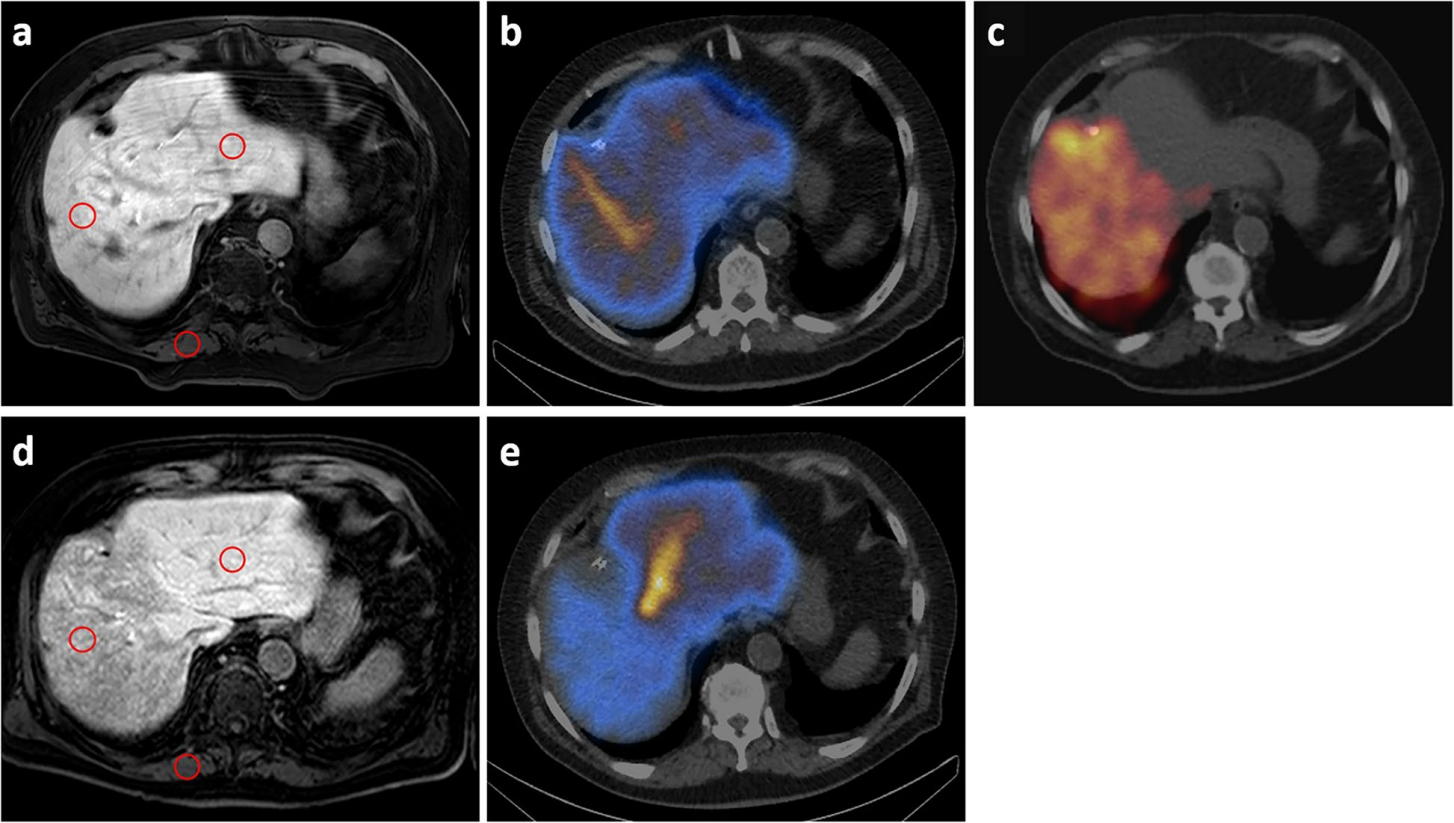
Case example of measurement of gadolinium ethoxy-benzyl diethylenetriamine pentaacetic acid (Gd-EOB-DTPA) uptake after radioembolisation. Regions of interest are placed in the left and right hepatic lobes (red circles), the spleen (not shown), and in the muscle in T1-weighted images obtained 20 min after Gd-EOB-DTPA, before (a) (baseline) and after RE (d) (follow-up). The measured mean signal intensities are used to calculate Gd-EOB-DTPA uptake according to Eqs. 1 and 2 (see the “Methods” section). The mean values at baseline (a) in RLL/LLL: LSR 1.9/1.9; LMR 1.8/1.8. The mean values measured at follow-up (d) in RLL/LLL: LSR 1.6/2.4; LMR 1.9/2.9. c The distribution of Y-90 microspheres in the right hepatic lobe in Bremsstrahlung SPECT/CT. b Baseline and e follow-up show the corresponding HBS SPECT/CT. HBS, Hepatobillary scintigraphy; LLL, Left liver lobe; LMR, Liver-to-muscle ratio; LSR, Liver-to-spleen ratio; RLL, Right liver lobe; SPECT/CT, Single-photon emission computed tomography/computed tomography
